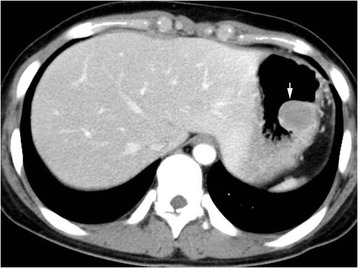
Abdominal CT scan. Abdominal CT scan shows a polypoid, submucosal mass (arrow) located in the distal body of the stomach
